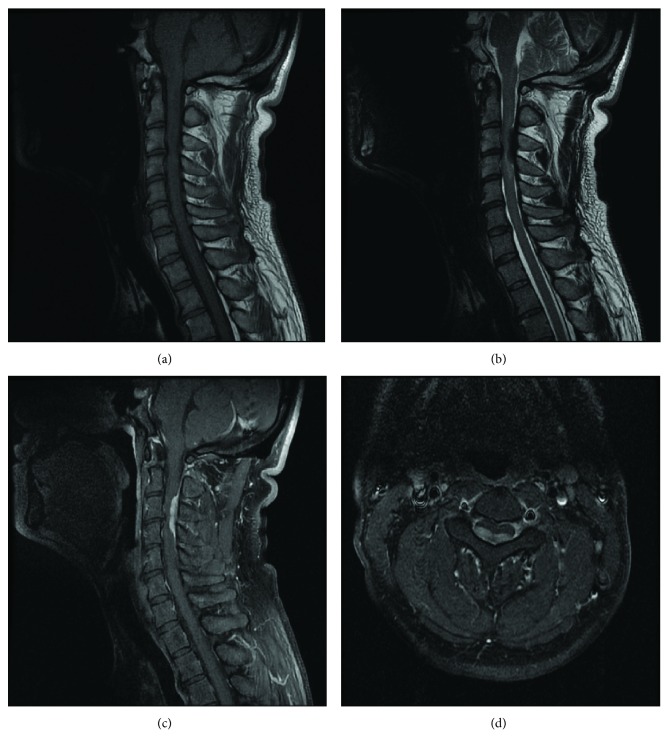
(a, b) MRI of the cervical spine showed IDEM mass, with low intensity in the T2-weighted imaging and isointensity in the T1-weighted imaging at the C3-4 levels. (c, d) The marked enhancement of the mass and a dural tail sign after intravenous admission of Gd-DTPA.
